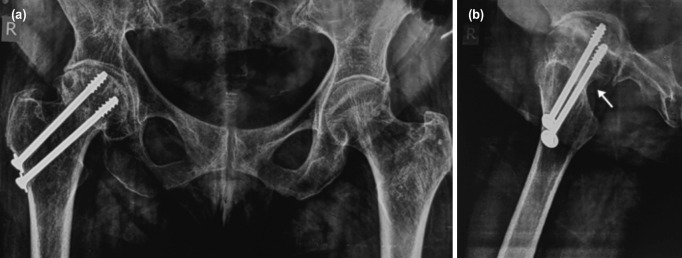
Pre-operative anteroposterior radiograph of both hips (a) with collapse femoral head, implant in situ and screw cut out and lateral radiograph of right hip (b) with screw abutment marked with white arrow at inferior femoral neck region.
